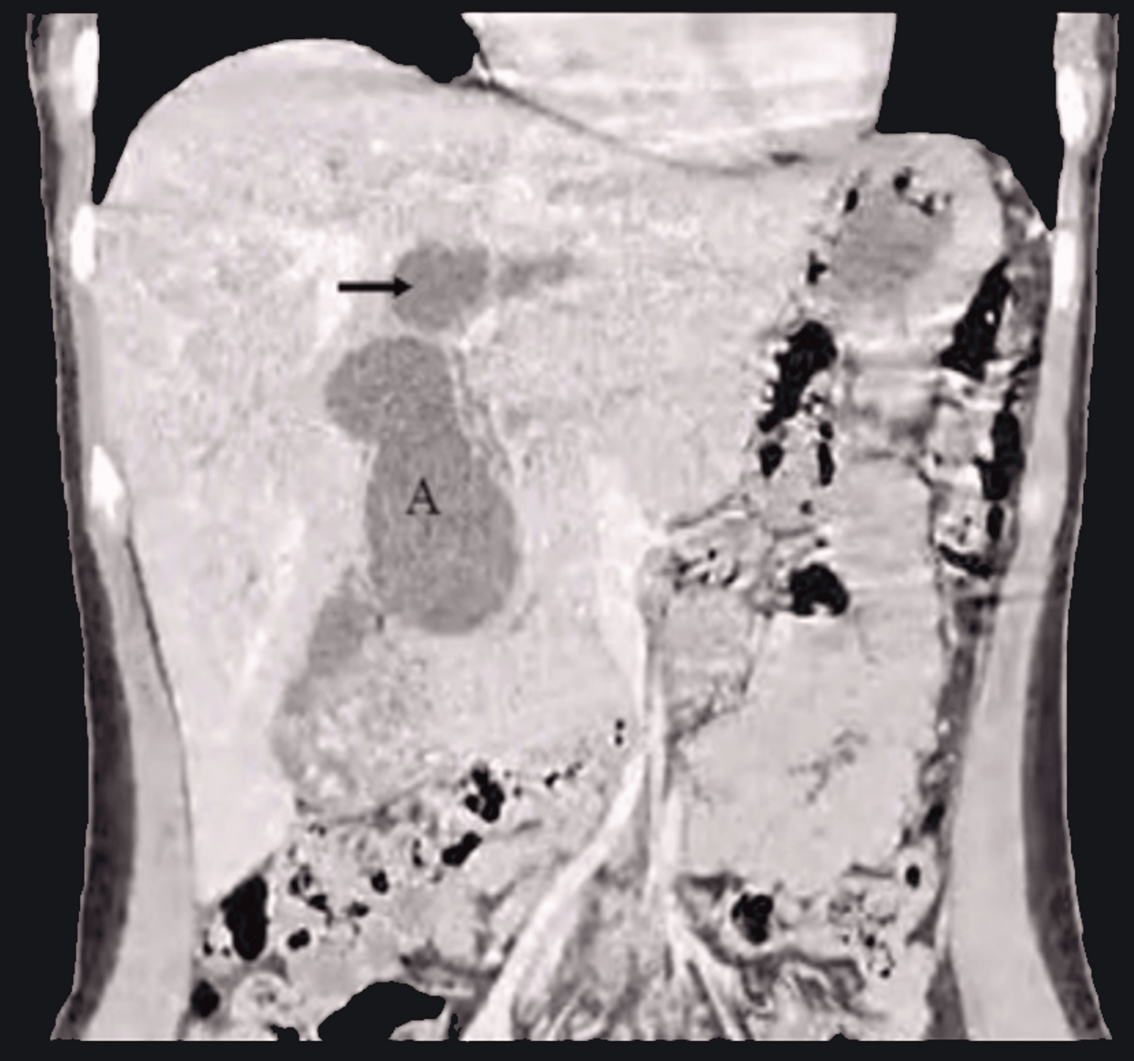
Contrast-Enhanced Abdominal Computed Tomography This coronal thoracoabdominal section shows both extrahepatic and intrahepatic bile ducts with significant dilation. The dilated common bile duct (A) is observed from its supraduodenal portion to the common hepatic duct (arrow), along with the left and right hepatic ducts.
